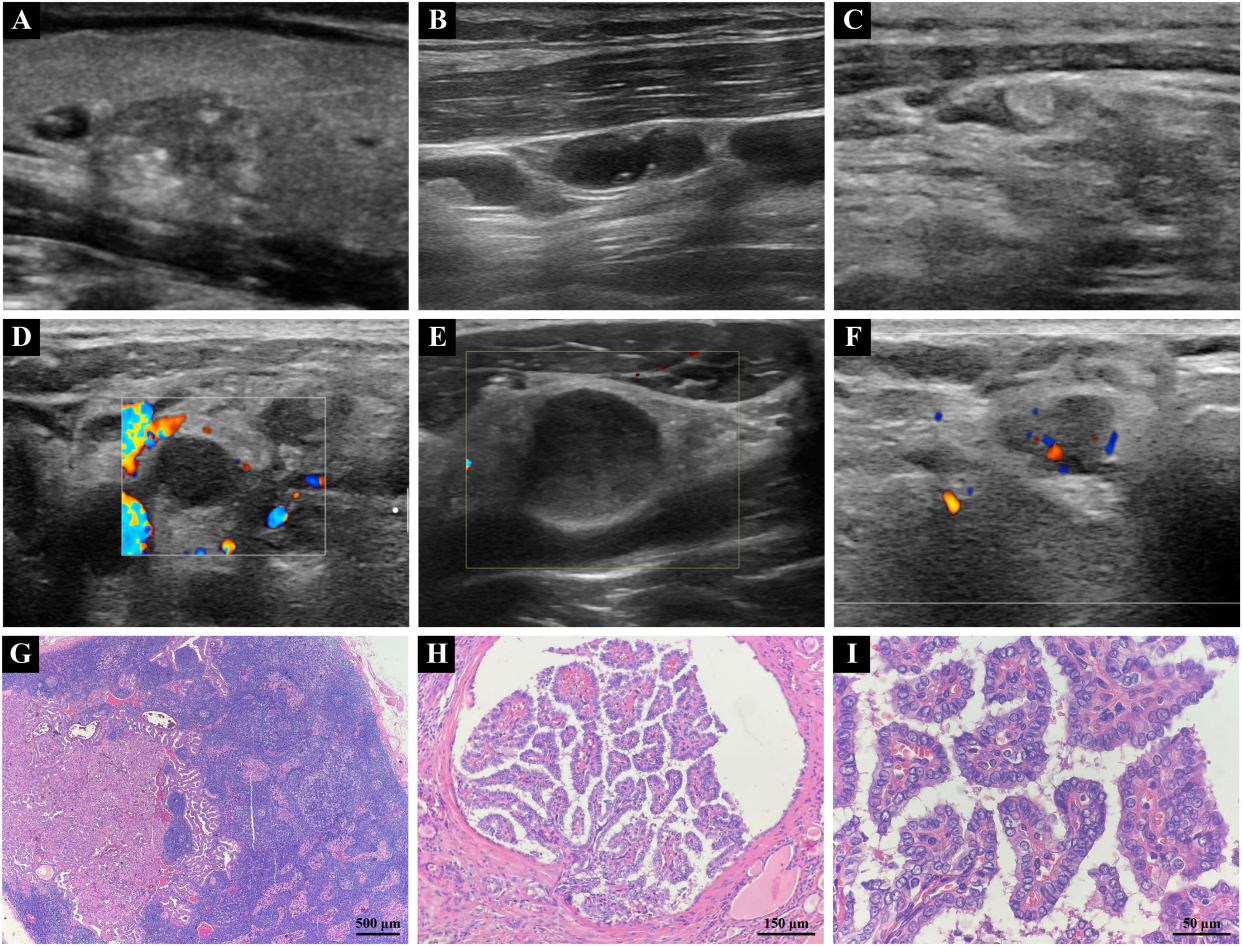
(A) Two-dimensional grayscale ultrasound image of PTC. (B) Typical ultrasonographic features of metastatic lymph node in PTC, showing cystic changes and punctate echogenic foci. (C) Typical ultrasonographic features of metastatic lymph nodes in PTC, demonstrating hyperechoic masses. (D) Atypical ultrasonographic features of metastatic lymph node in PTC, Lymph nodes are round or roundish. (E) Atypical ultrasonographic features of metastatic lymph node in PTC, Loss or eccentricity of the lymph node hilum or poor demarcation of the corticomedullary stroma. (F) Atypical ultrasonographic features of metastatic lymph node in PTC, Irregular or blurred borders. (G) Metastasis of PTC tumor identified within a cervical lymph node (4X). (H) PTC tumor exhibiting a branching papillary structure with invasive growth within thyroid tissue (20X). (I) PTC tumor cells showing enlargement, crowding, and overlapping, with ground-glass nuclei and visible nuclear grooves (60X). (PTC, papillary thyroid carcinoma).
